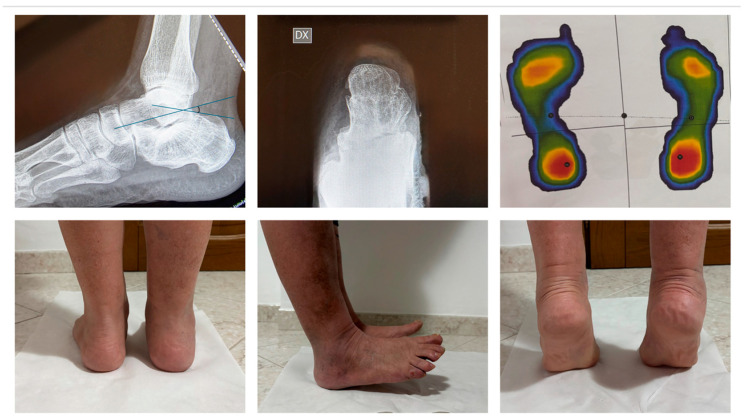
65-year-old male patient at 18 months of F.U.: good clinical and radiographical results. Böhler’s angle was 32.1°. The baropodometric evaluation showed overlapping pressure areas between the two feet.
